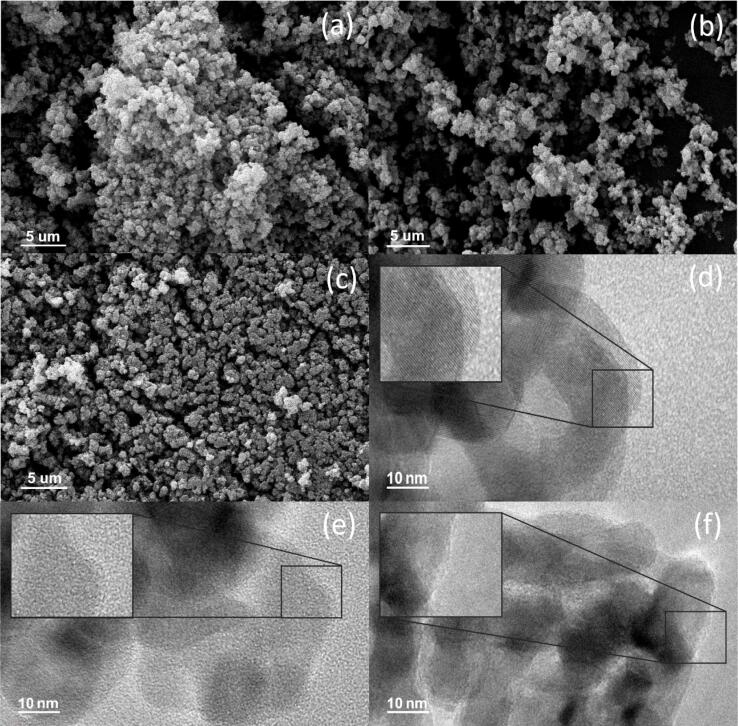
(a-c) SEM images of (a) TiO_2_; (b) ultrasonic cleaner-TiO_2_; (c) ultrasonic horn-TiO_2_. And (d-f) TEM images of (d) TiO_2_; (e) ultrasonic cleaner-TiO_2_; (f) ultrasonic horn-TiO_2_.
